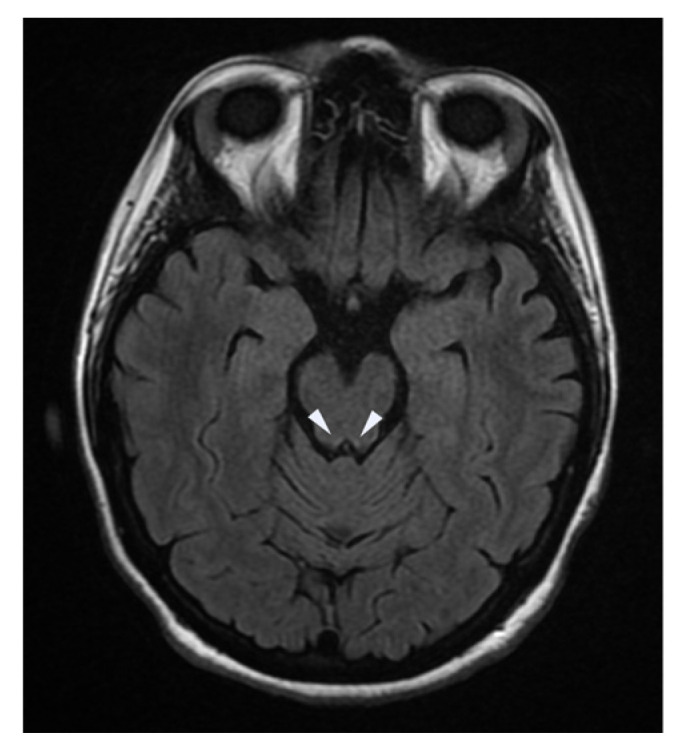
FLAIR axial view of the colliculi.
